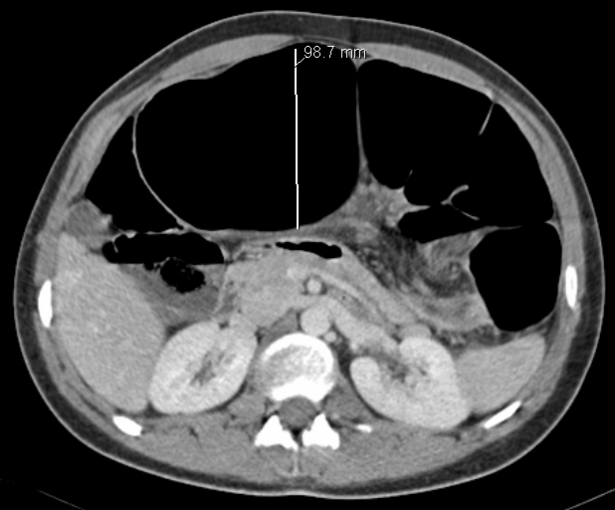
Abdominal CT provides a differential of a colo-colic intussusception or volvulus.
